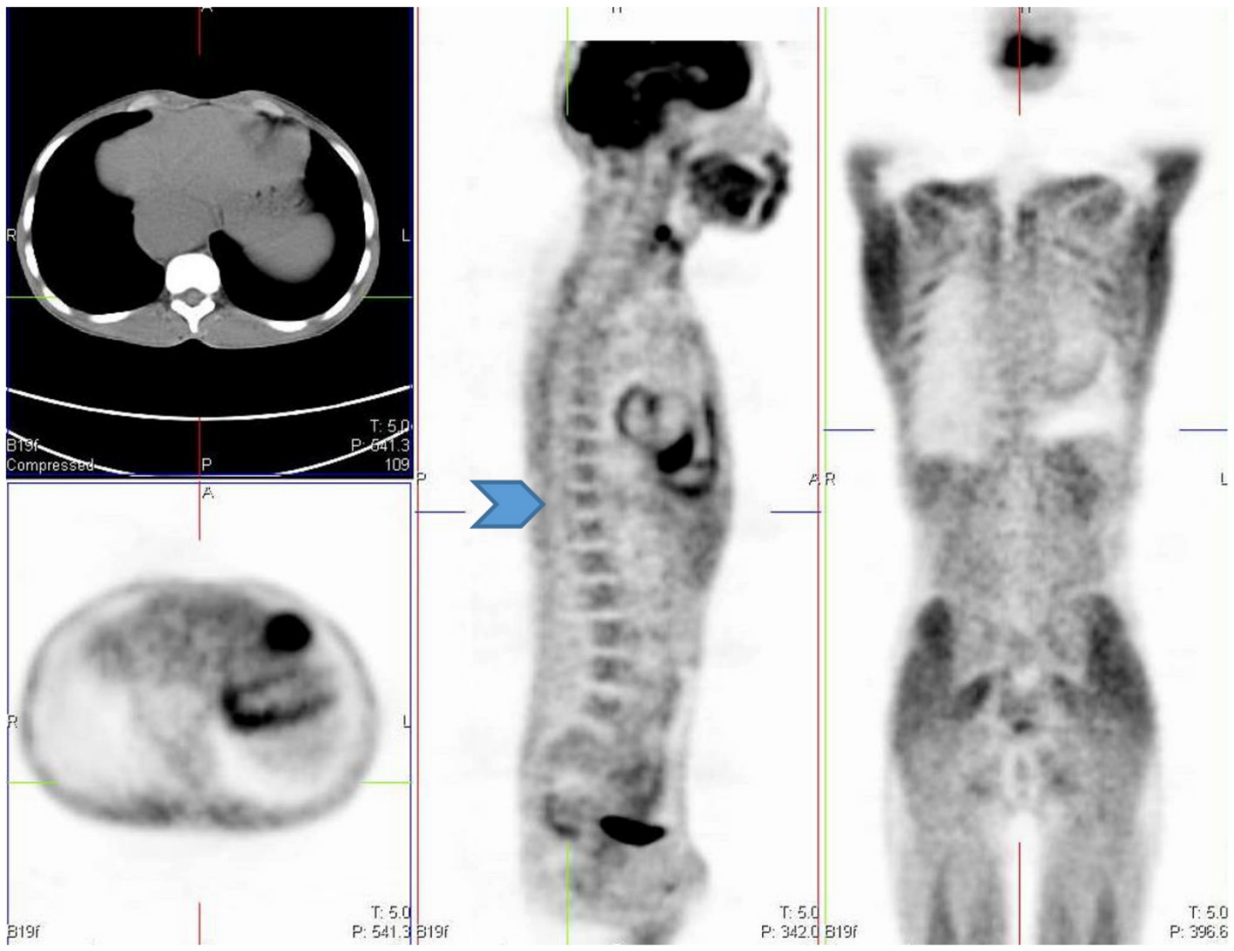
12 year old female referred for evaluation of active vasculitis. Incidental finding of linear uptake in the conus medullaris at T11-L1 level is physiological (Blue arrowhead). Also note the diffuse uptake in the muscles of the chest and lower limb due to playing sport the day before the injection. Other causes of diffuse muscle uptake may include high glucose level prior to injection of FDG.
